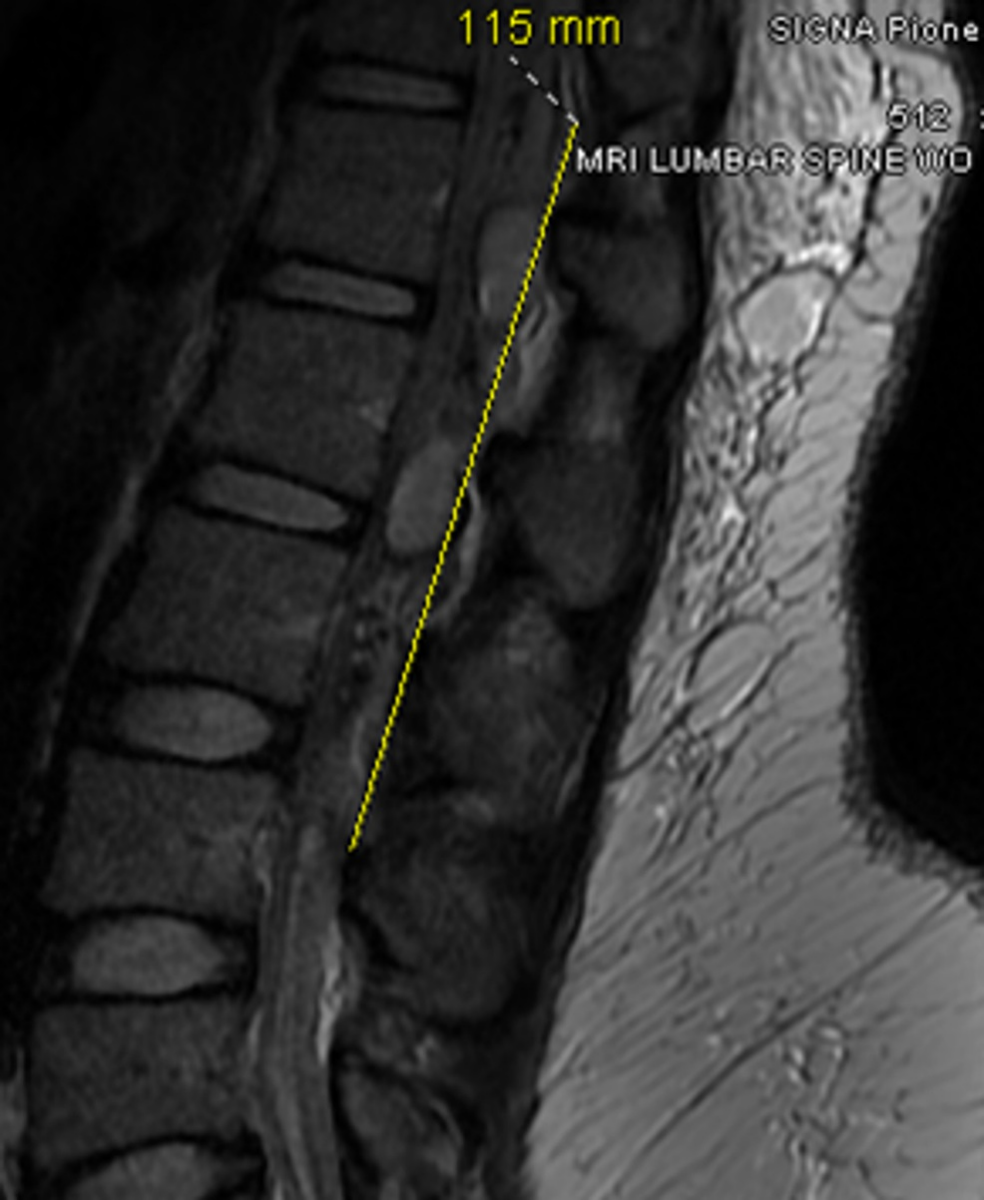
Sagittal T2 MRI of the lumbar spine without contrast showing the craniocaudal extension of the hematoma of 11.5 cm extending from T12/L1 to L4 level MRI, Magnetic resonance imaging.
